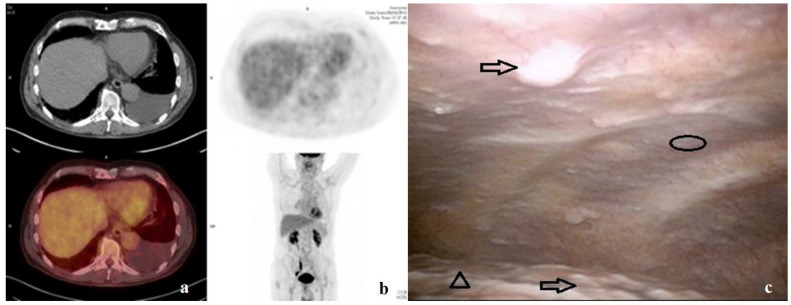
Direct visualization of the pleural cavity in patient with non-specific exudative pleural effusion. (a) CT-scan showing pleural effusion without macroscopic pleural abnormalities. (b) Normal ^18^FDG-CT scan. (c) Pleural cavity with multiple nodules (arrow) on the parietal pleura (circle) and on the surface of the lung (arrowhead).
